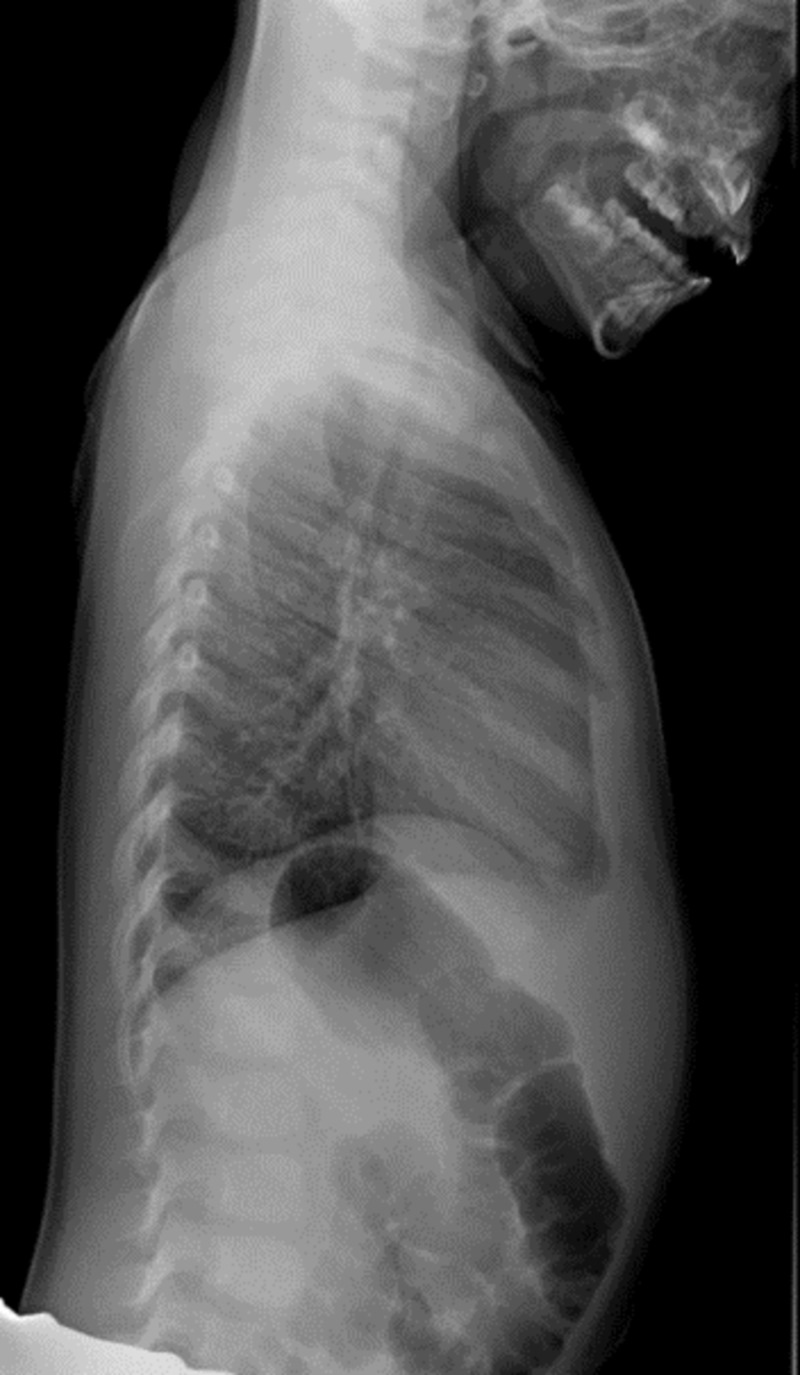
Chest radiograph in the lateral view This chest radiograph in the lateral view shows no evidence of any pulmonary infiltrate or foreign body.
